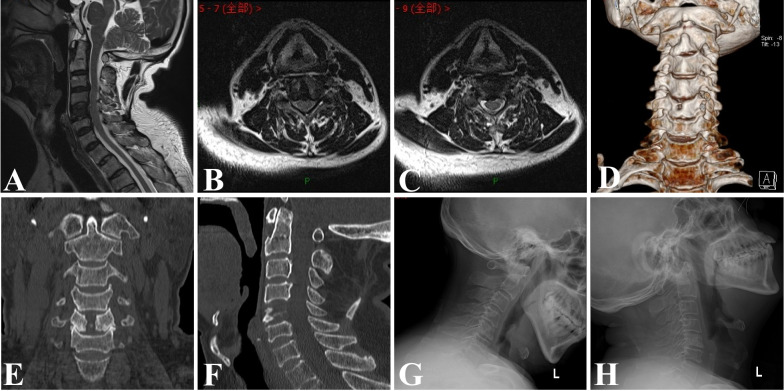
At 6 months after surgery, sagittal and axial MRI showed adequate decompression of the spinal cord (A-C). The CT-3D reconstruction and coronal images showed good healing of the bony channels without collapsed fractures of the drilled vertebral body (D, E), and no displacement of the autologous bone was observed in the sagittal position (F). The power position X-ray showed no loss of vertebral height or cervical instability (G, H)
